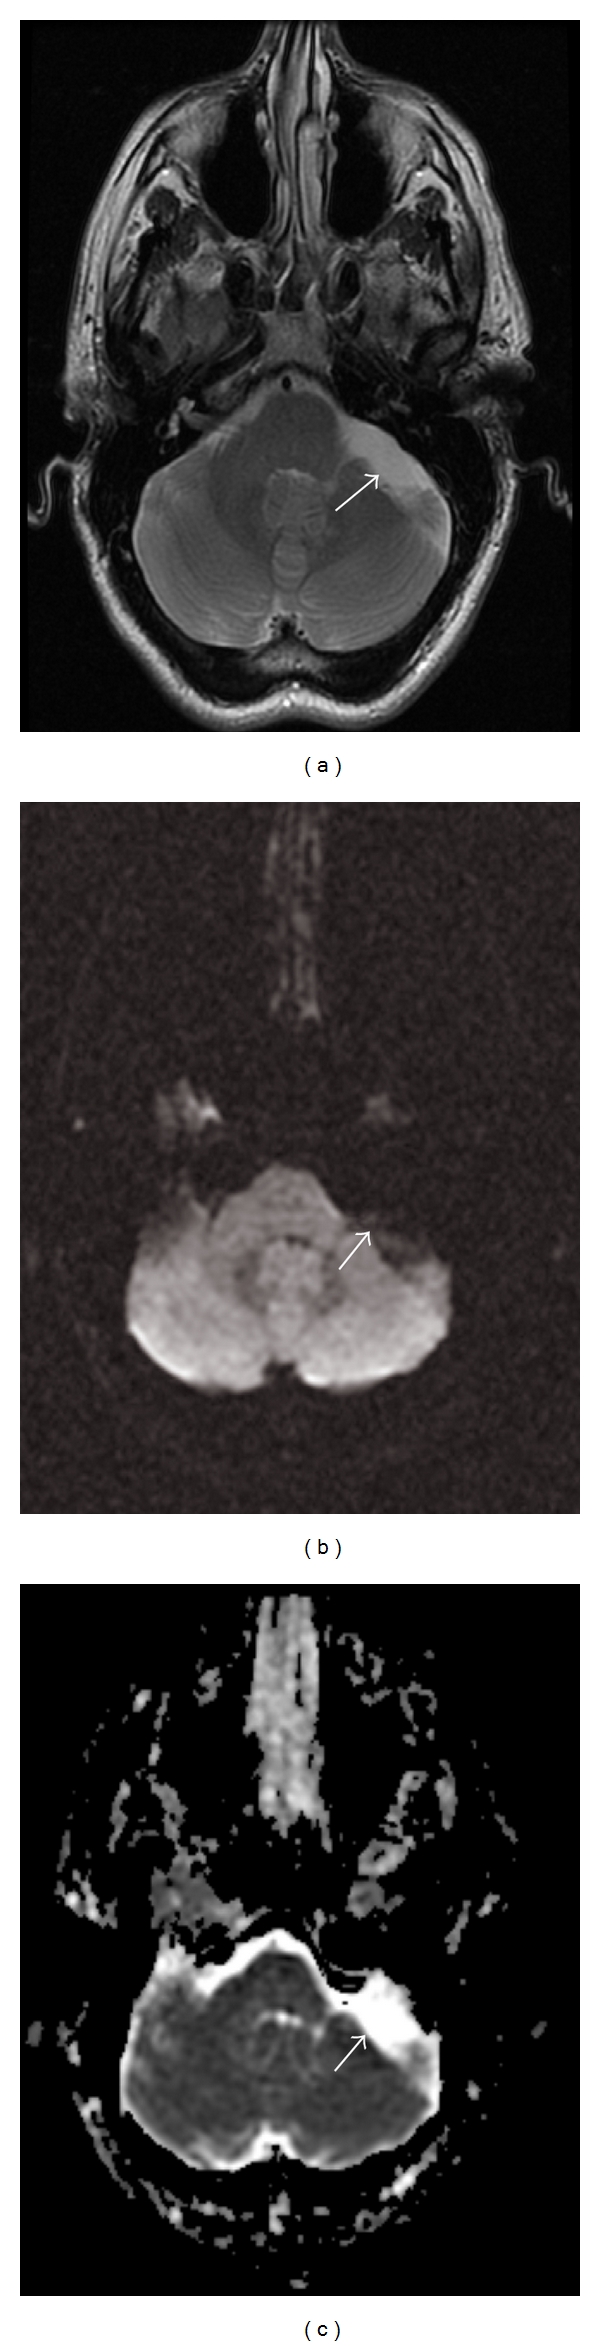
A 32-year-old man with a metastatic melanoma with an incidentally detected left cerebellopontine angle arachnoid cyst. (a) Axial Fast Spin Echo T2 sequence. T2 hyperintensity is seen in the cerebellopontine angle mass (arrow). (b) DWI sequence. The mass is dark on diffusion-weighted imaging. (c) ADC map. Signal hyperintensity in the lesion on the ADC map (arrow) is present. These findings are consistent with a cyst and exclude other lesions such as an epidermoid.
